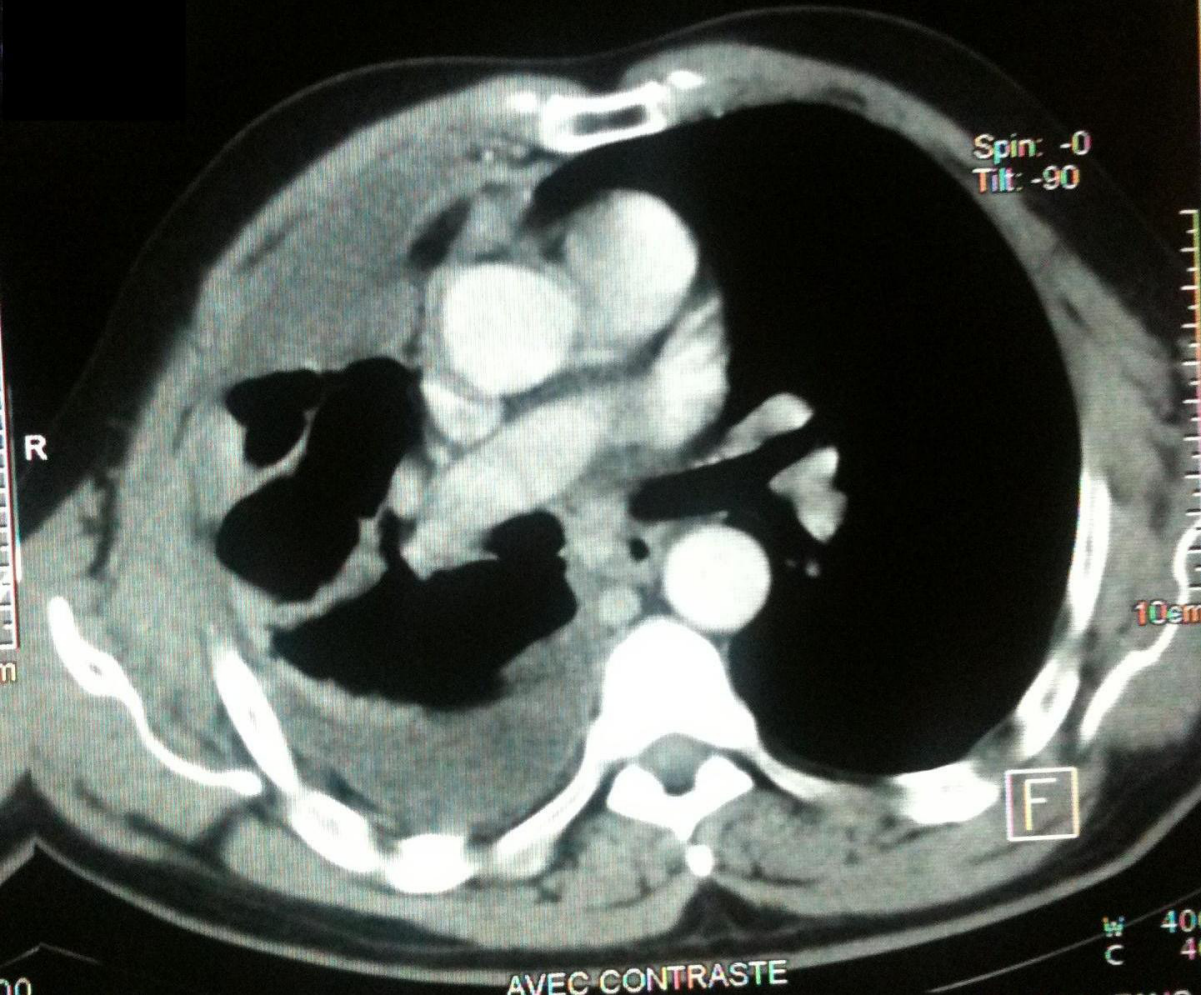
Selected sections of a conventional computed tomography scan of the chest. The scan shows a right-sided cavity with thick and irregular walls in the right lower lobe, pleural effusion, and a partially collapsed right lung.
